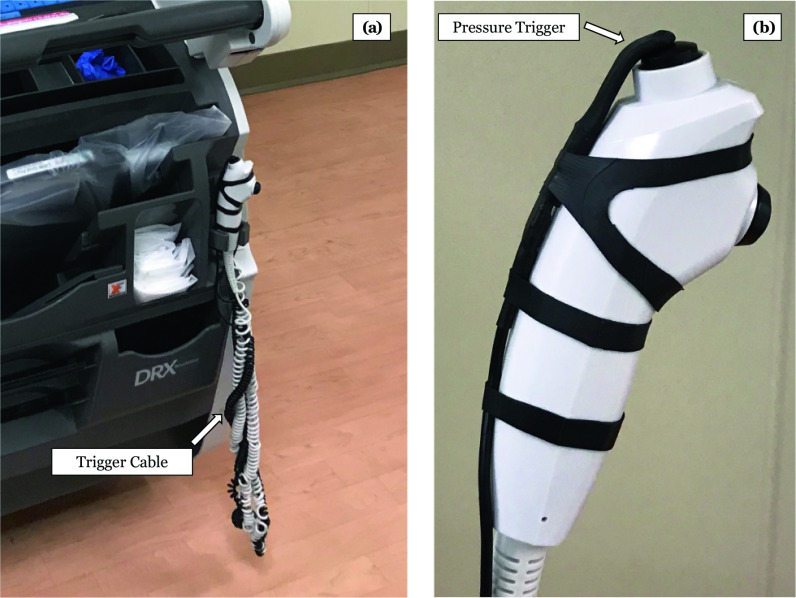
a Initial hardware implementation for simultaneous triggering of photography acquisition. The pressure sensor is attached to the portable radiography machine trigger. b The camera trigger cable is strung along the radiography trigger cable showing how this can be tangled
